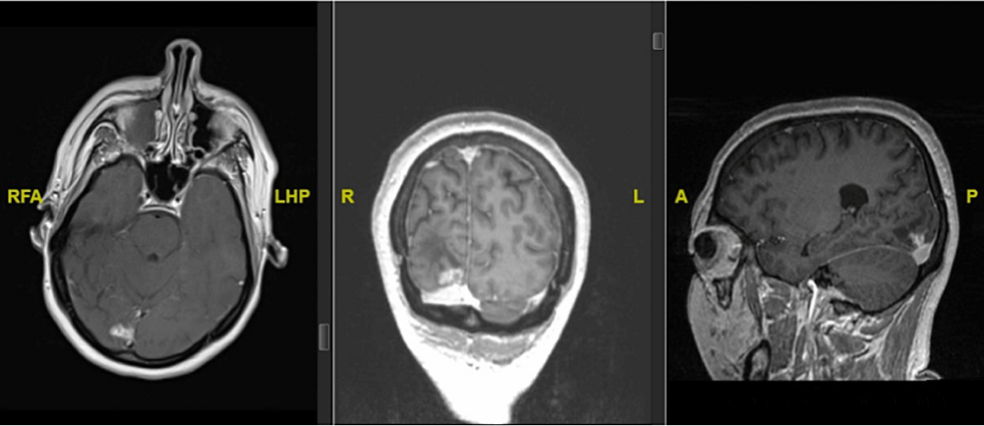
(From left to right) Axial, coronal, and sagittal views of MRI brain with contrast demonstrating a previously treated arteriovenous malformation in the right lingual gyrus with evidence of gliosis RFA, R: right. LHP, L: left. A: anterior. P: posterior LHP: left horizontal position
